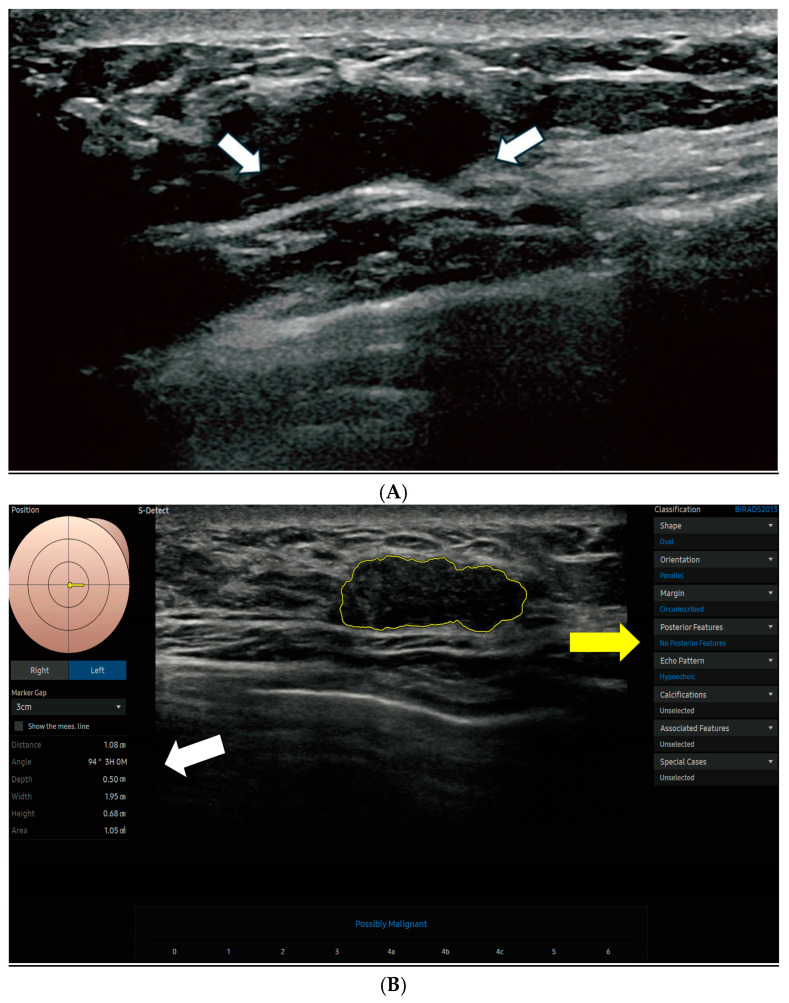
Breast ultrasound and S-Detect AI analysis in a 44-year-old woman with a BI-RADS 4A lesion. (A) B-mode ultrasound image of the left breast demonstrates a hypoechoic lesion (arrows), initially categorized as BI-RADS 4A by the interpreting radiologist. (B) The S-Detect AI delineates the lesion margins and provides associated quantitative size parameters (white arrow): distance, 1.08 cm; angle, 94°; depth, 0.50 cm; width, 1.95 cm; height, 0.68 cm; and area, 1.05 cm^2^. The AI-generated BI-RADS lexicon assessment (yellow arrow) characterizes the lesion as oval, parallel-oriented, circumscribed, hypoechoic, and without posterior acoustic features.
